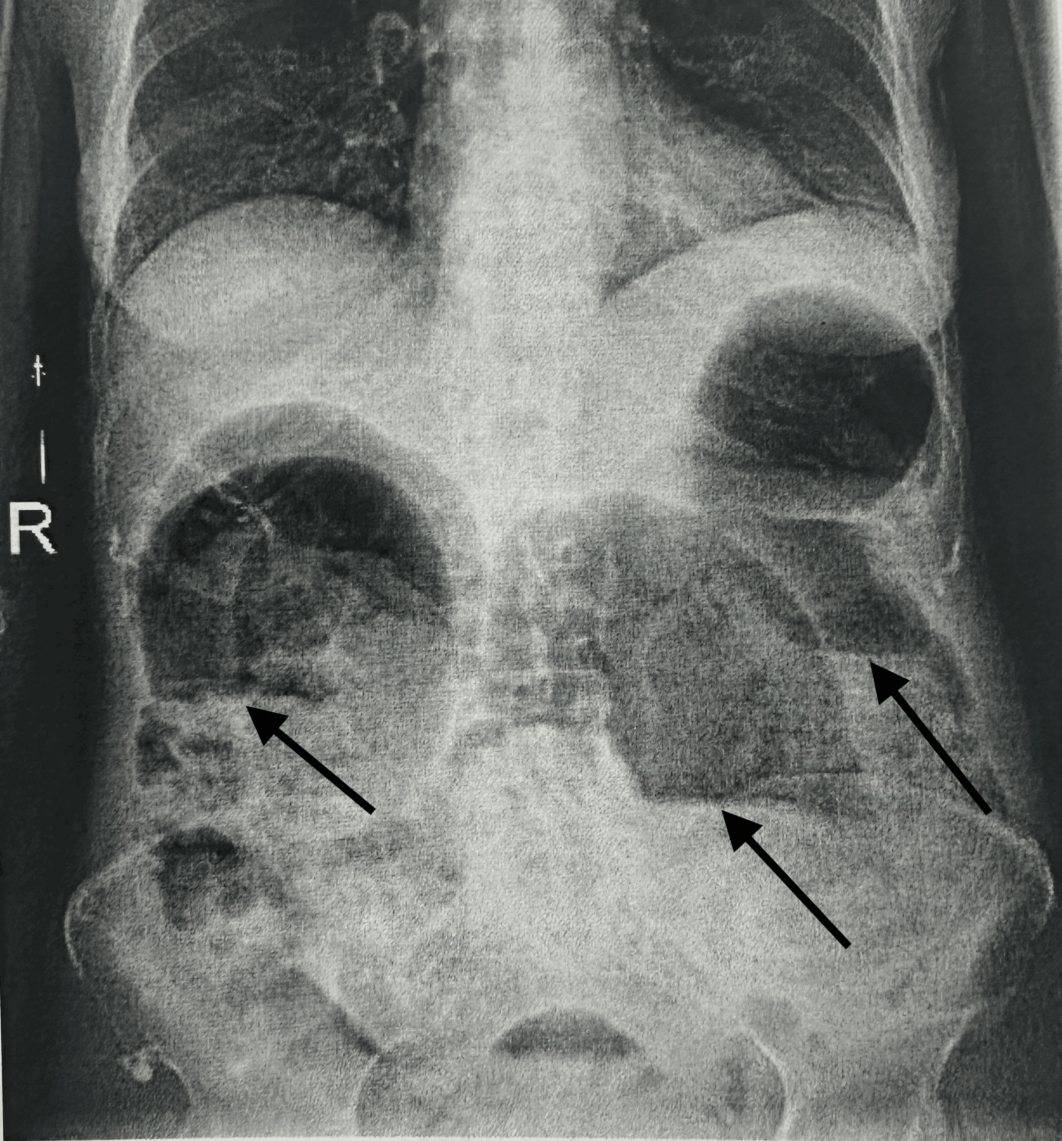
Abdominal radiograph demonstrates multiple centrally located, dilated bowel loops with visible air-fluid levels. Visible air-fluid levels indicated by arrows.
